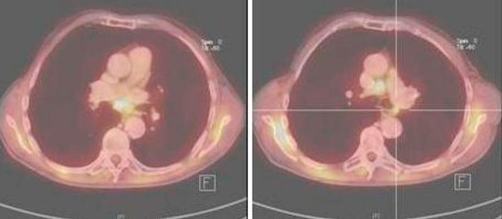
Further uptake was detected in precarinal (SUVmax: 6.4), infracarinal (SUVmax: 6.4) and paraaortal (SUVmax: 4.0) lymph nodes as well in a lymph node at the left main bronchus (SUVmax: 4,0), correspond to N3 disease.
